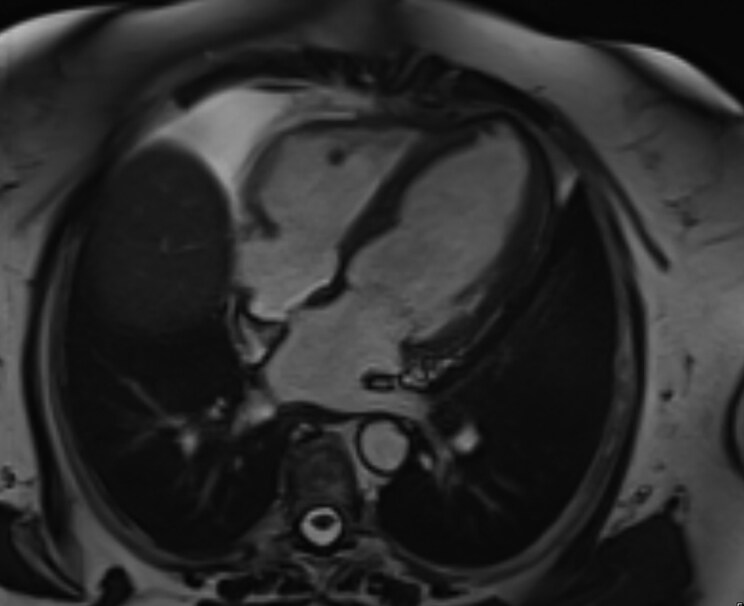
Four-chamber CINE cardiac MRI at 3-month follow-up demonstrating continued but improved myocardial inflammation and oedema compared to prior imaging, with no new non-ischaemic fibrosis.
